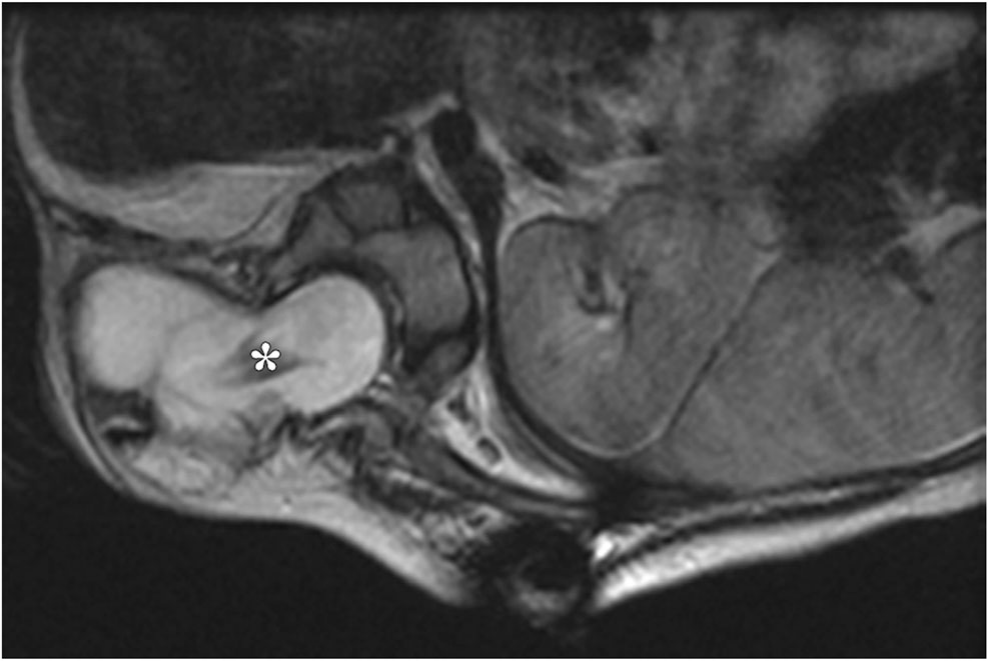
Axial T2-weighted MRI in a 1-month-old infant with a right posterior paravertebral mass. Non-contrast axial T2-weighted MRI identifies a right lateral myelomeningocele. Besides the herniated meninges, a neural placode (white star), which was not seen on CT (not shown), is also seen on the MRI, thus confirming this to be a lateral thoracic myelomeningocele
